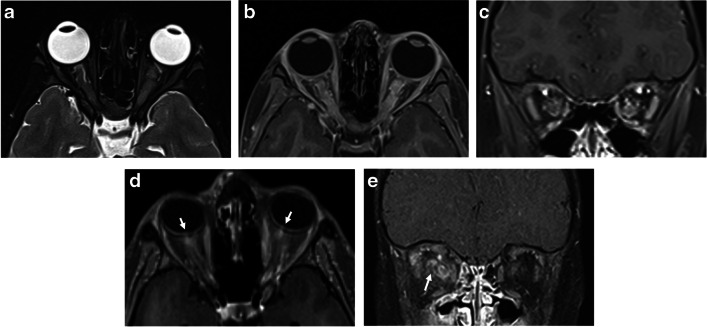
Optic neuritis in three children diagnosed with myelin oligodendrocyte glycoprotein antibody-associated disease. a-c Magnetic resonance (MR) images of the orbits obtained from a 5-year-old girl presented with encephalopathy and bilateral optic neuritis. a Axial fat-suppressed T2-weighted image shows bilateral longitudinally extensive optic neuritis with T2-hyperintensity along the intra-orbital segments of the optic nerves. b Axial and c coronal fat-suppressed contrast-enhanced T1-weighted images show enhancement along the involved segments, as well as of the surrounding perineural tissues. d Axial fat-suppressed contrast-enhanced T1-weighted MR image of the orbits obtained from a 17-year-old girl presented with diplopia and left side paresthesia shows enhancement and swelling of the bilateral optic nerve heads (*arrows*). e Coronal fat-suppressed contrast-enhanced T1-weighted MR image of the orbits obtained from a 28-month-old boy presented with encephalopathy shows bilateral asymmetric optic neuritis, more prominent on the right side, with enhancement of the optic nerve, optic nerve sheath, and perineural tissues (*arrow*)
